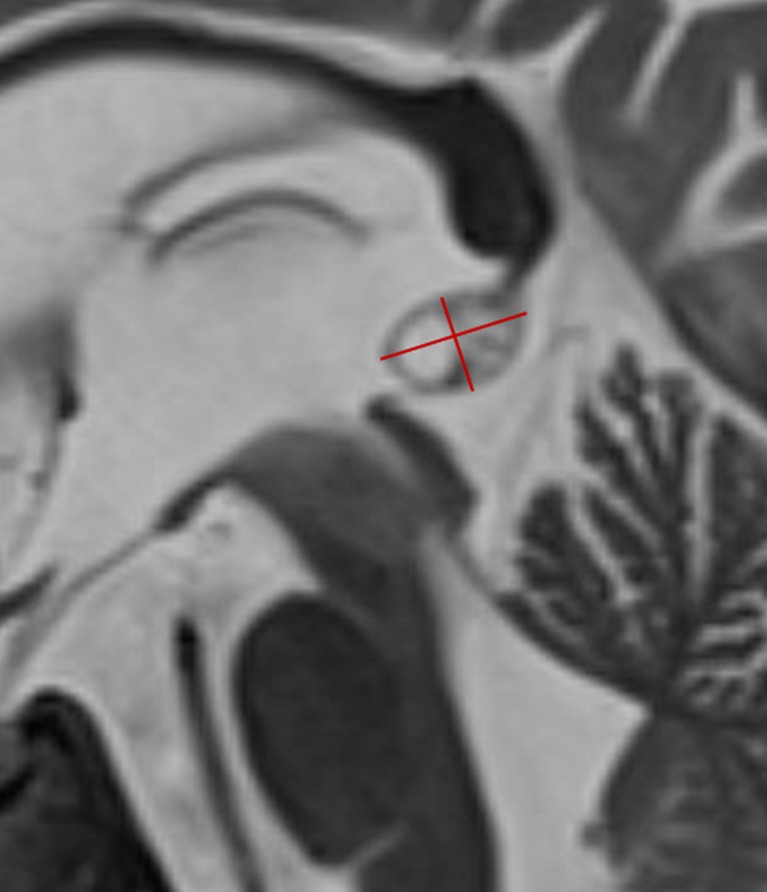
The largest anteroposterior (width) and craniocaudal (height) diameters of the pineal gland were measured as shown in this sagittal T2-weighted image
